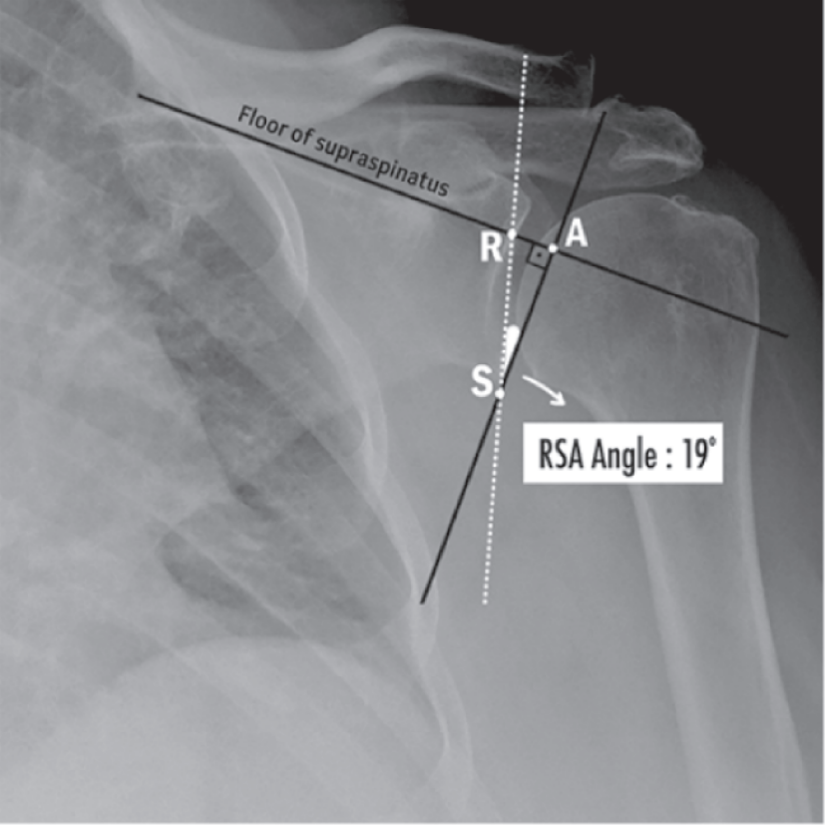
Preoperative X-ray demonstrate measurement of the RSA angle in X-ray. RSA, Reverse shoulder arthroplasty.
